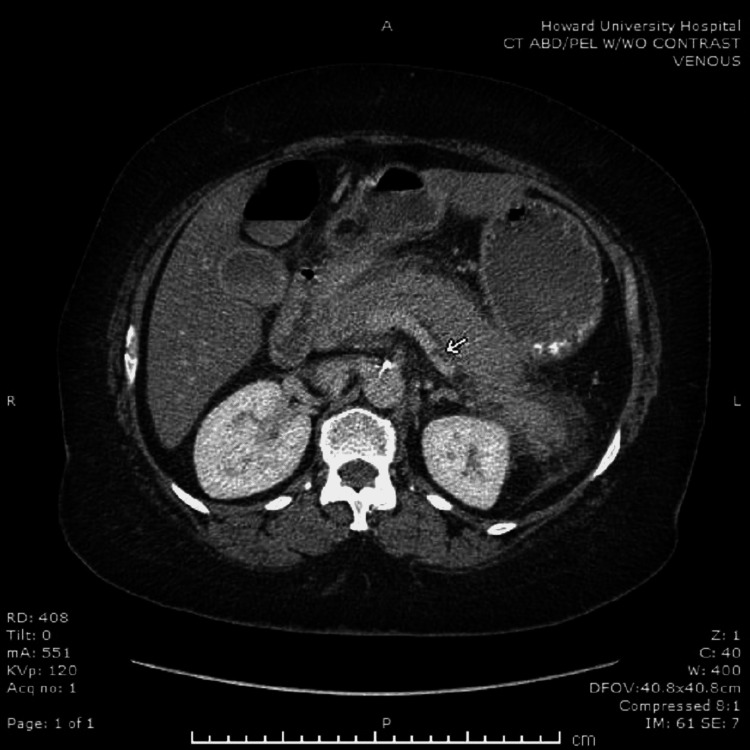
Abdominal CT scan without contrast showing thrombus in the splenic vein (white arrow)
